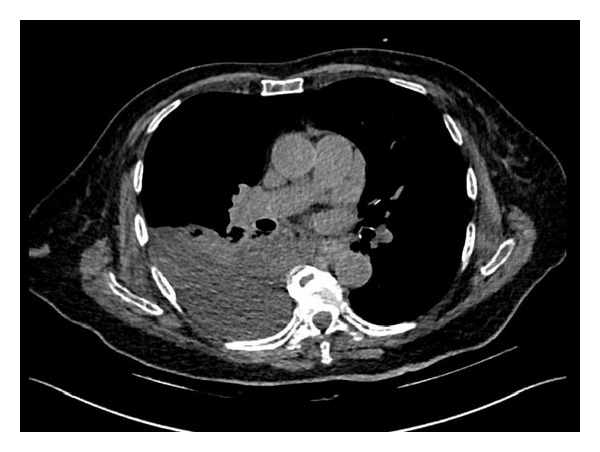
CT scan (MDCT, arterial with 40 mL Ultravist 370 and 5 min p.i. DLP 335 mGycm) of the thorax.
